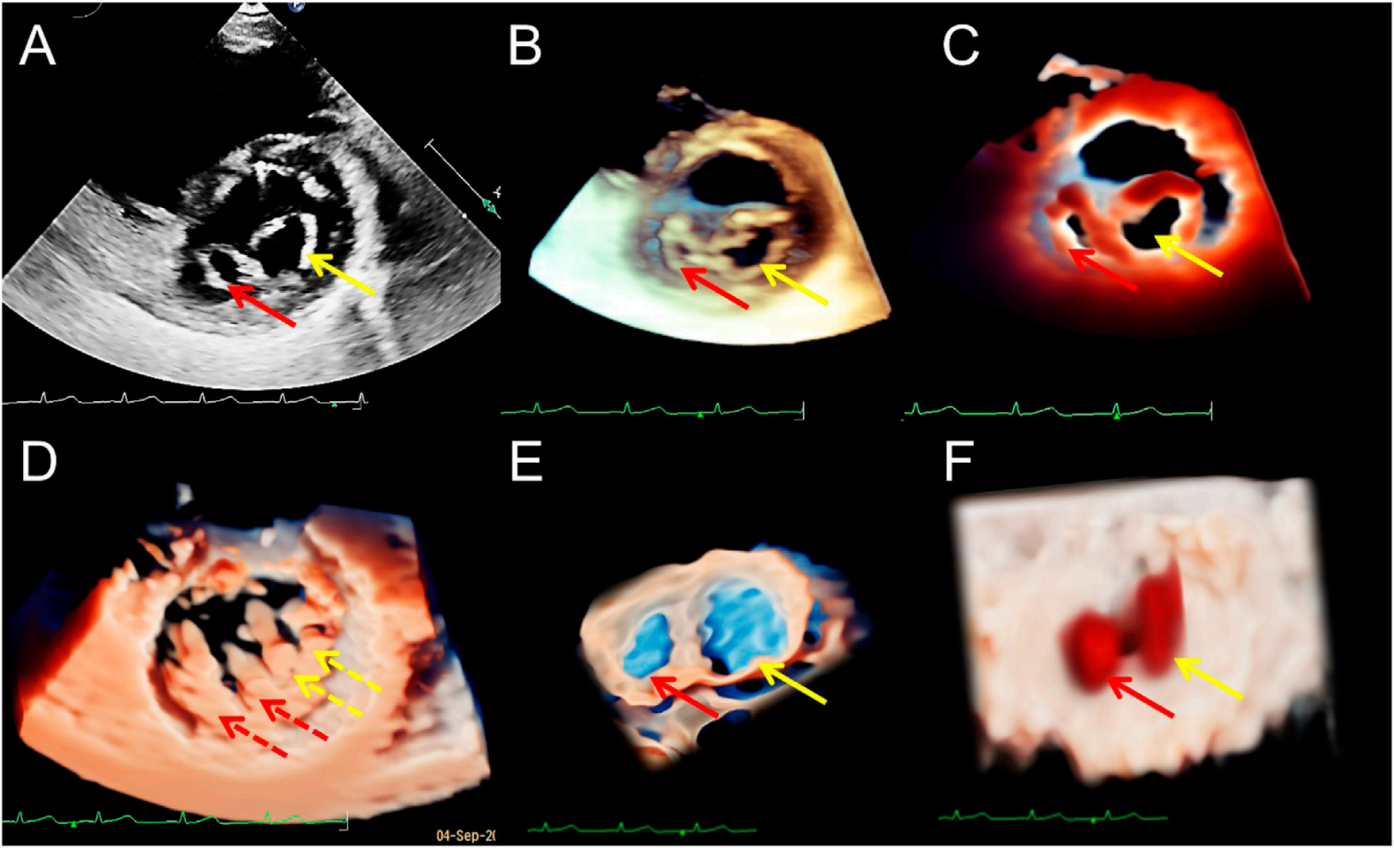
Transthoracic echocardiography of the short-axis view of the mitral valve (MV) showing the double orifice MV (arrows). (A). 2D display of asymmetric orifices; (B,C). Traditional 3D and TrueVue show the spatial shape of the orifices; (D). TrueVue shows the abnormal multiple papillary muscles and the tendons connected to them from the perspective of the left ventricle; the red dotted arrows represent the additional structure corresponding to the small orifice, and the yellow dotted arrows represents the additional structure corresponding to the large orifice. TrueVue Glass (E) plus color Doppler (F) directly visualizes the two orifices of the MV from the left atrium side (surgical view) and the two blood streams entering the left ventricle during diastole. The red and yellow solid line arrows in (A–C,E,F) indicate two asymmetric orifices of MV, respectively. 2D, two-dimensional; 3D, three-dimensional.
